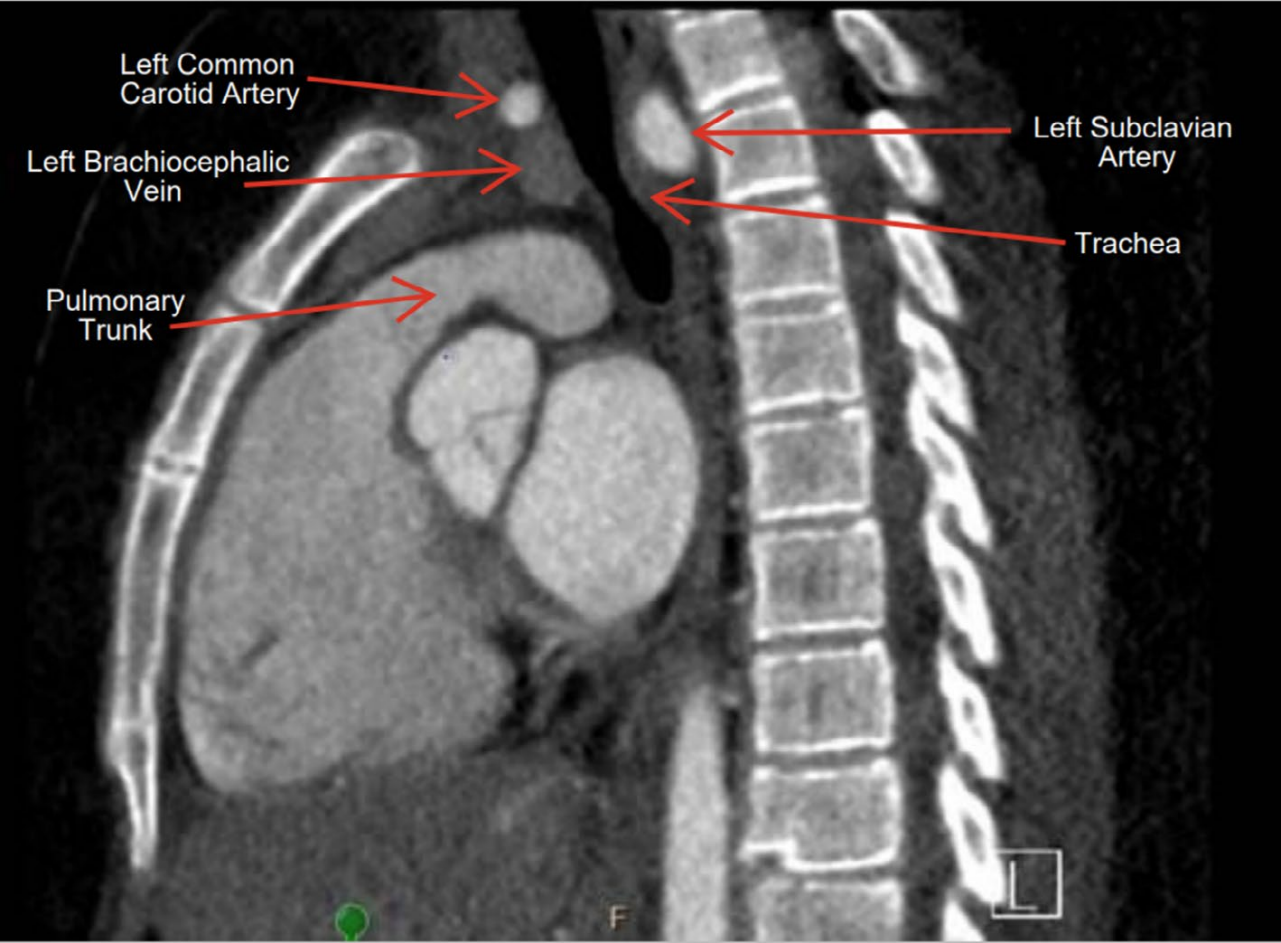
Computed tomography image showing fixed tracheal compression caused by the patient's double aortic arch.
